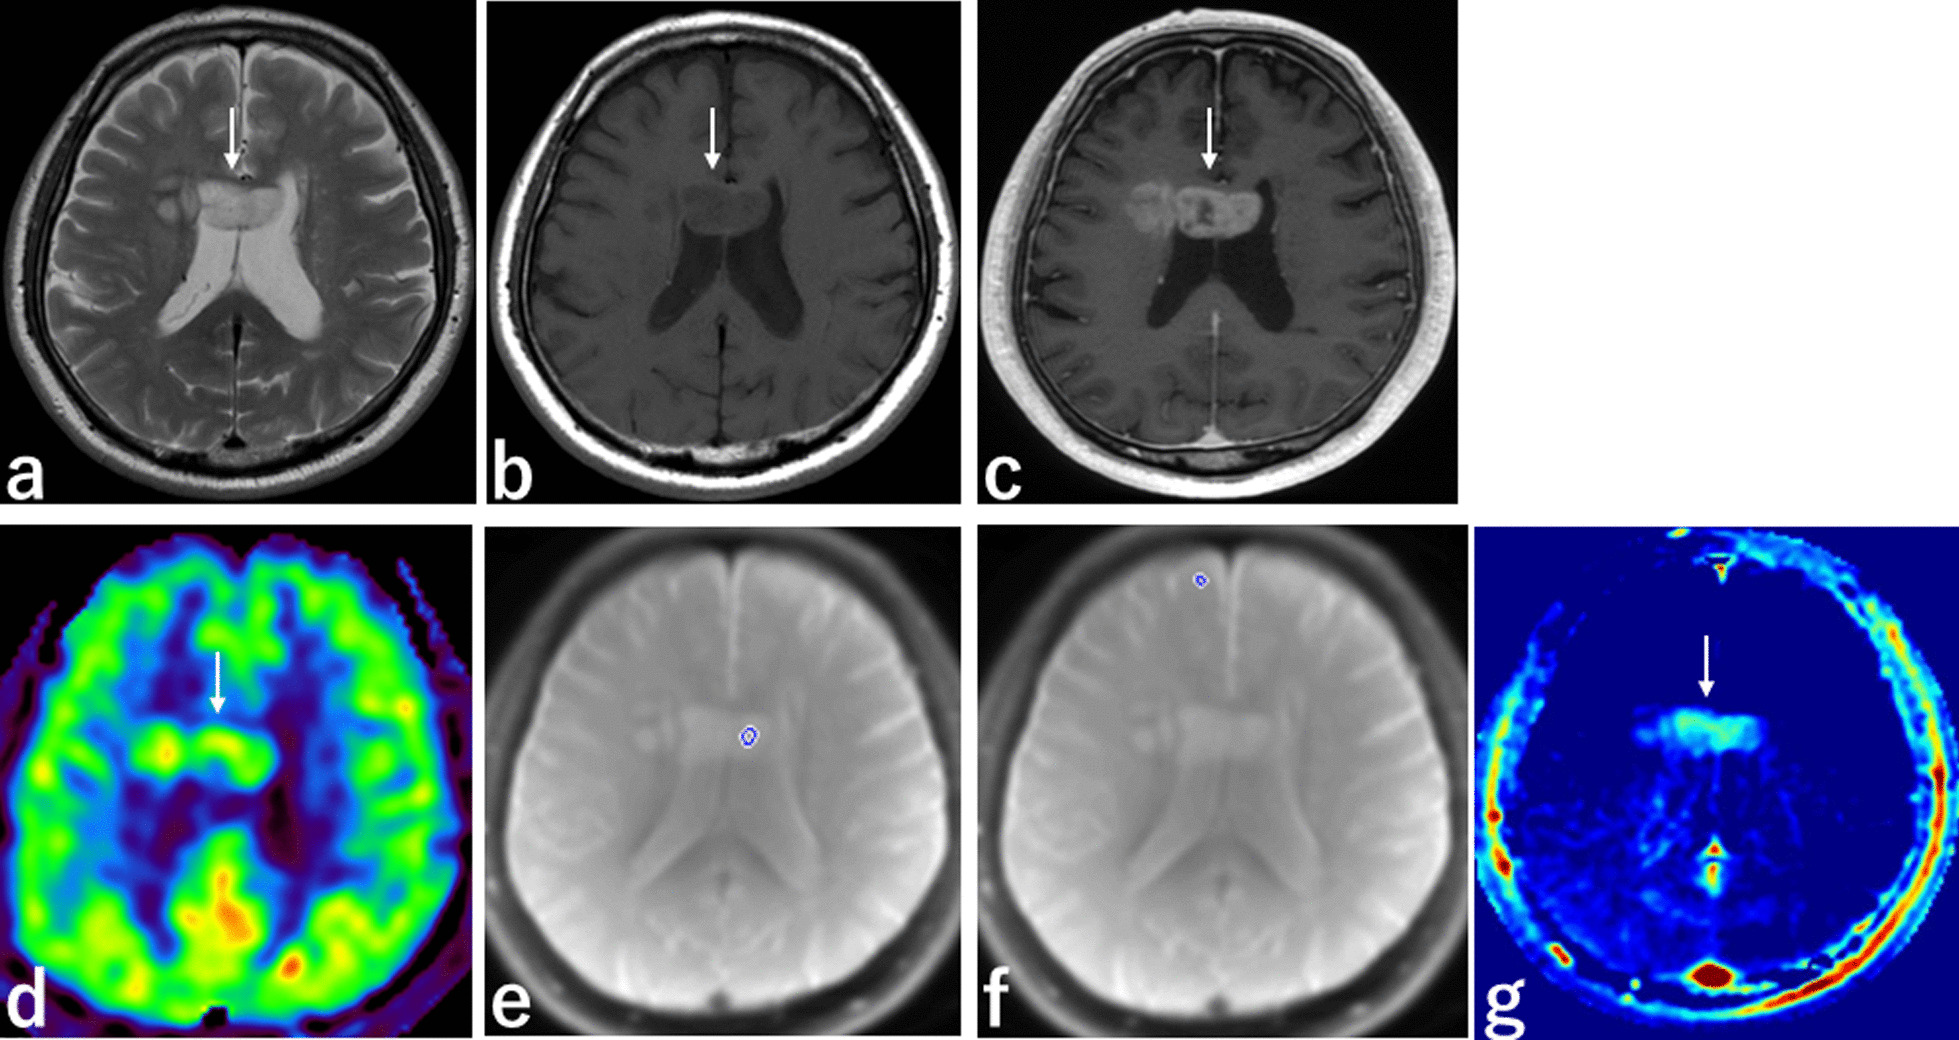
MRI from a 73-year-old man with GBM. a T2-weighted sequence, axial section, shows well-defined GBM with high SI in the body of the corpus callosum (white arrow). b T1-weighted sequence, axial section, shows well-defined GBM with low SI in the body of the corpus callosum (white arrow). c 3D contrast-enhanced T1 sequence, axial section, shows enhancement with a poor enhancement area in GBM (white arrow). d 3D ASL sequence, axial section, shows slightly high SI in GBM. e APT scout image, axial section, shows the ROI placed in the GBM avoiding the poor enhancement area that may represent a necrotic portion. f APT scout image, axial section, shows the ROI placed in normal-appearing tissue in right frontal lobe. g APT imaging shows high SI in GBM (white arrow). *GBM* glioblastoma multiforme, *SI* signal intensity, *ASL* arterial spin labelling, *APT* amide proton transfer
